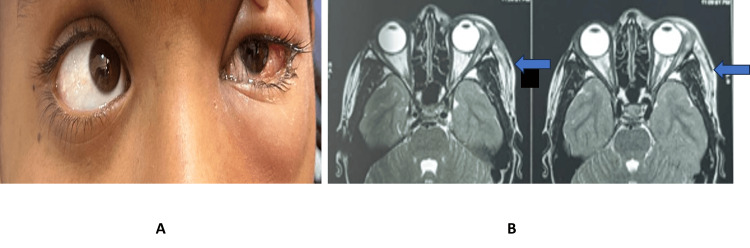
Clinical photograph and MRI findings in isolated LR palsy. (A) Left eye lateral rectus palsy. (B) MRI showing localized orbital abscess with orbital cellulitis with acute dacryoadenitis.
